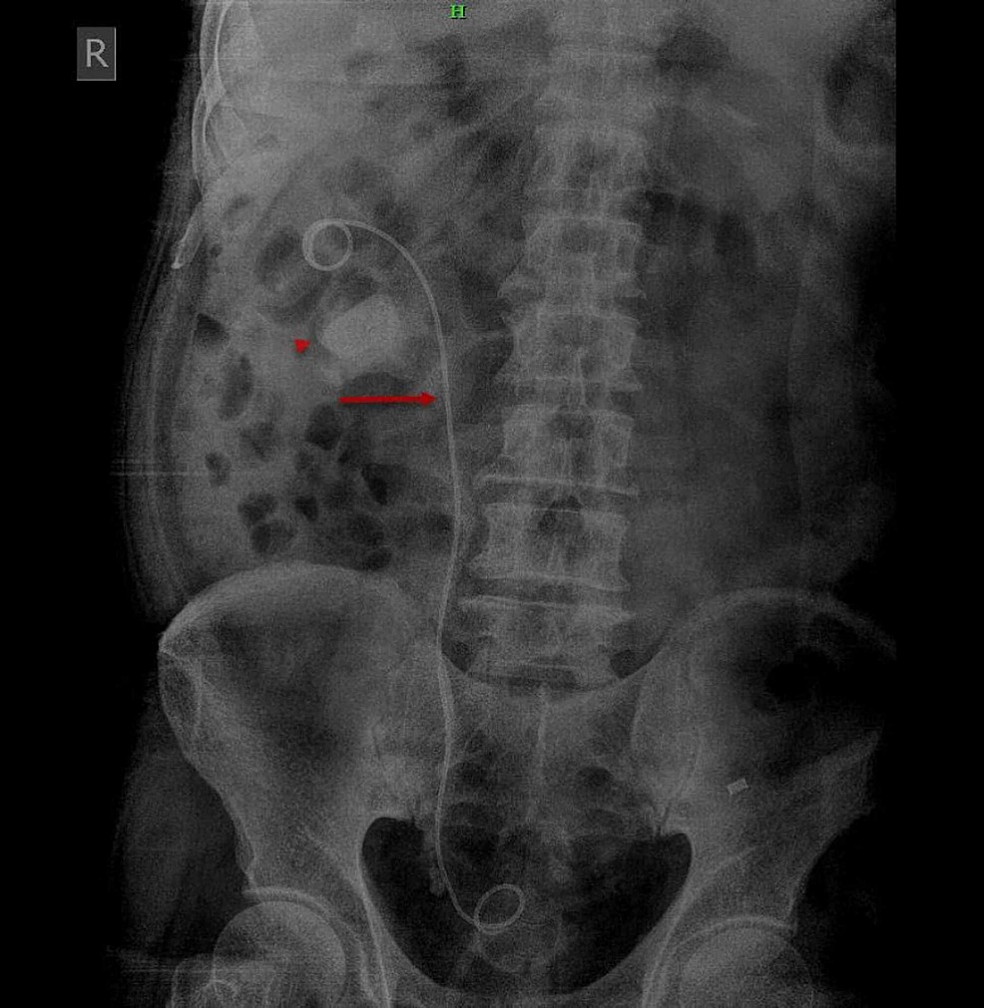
X-ray of kidney-ureter-bladder revealed a DJ stent on the right side (red arrows). A large radiodense calculus was noted on the right side (red arrowhead).
